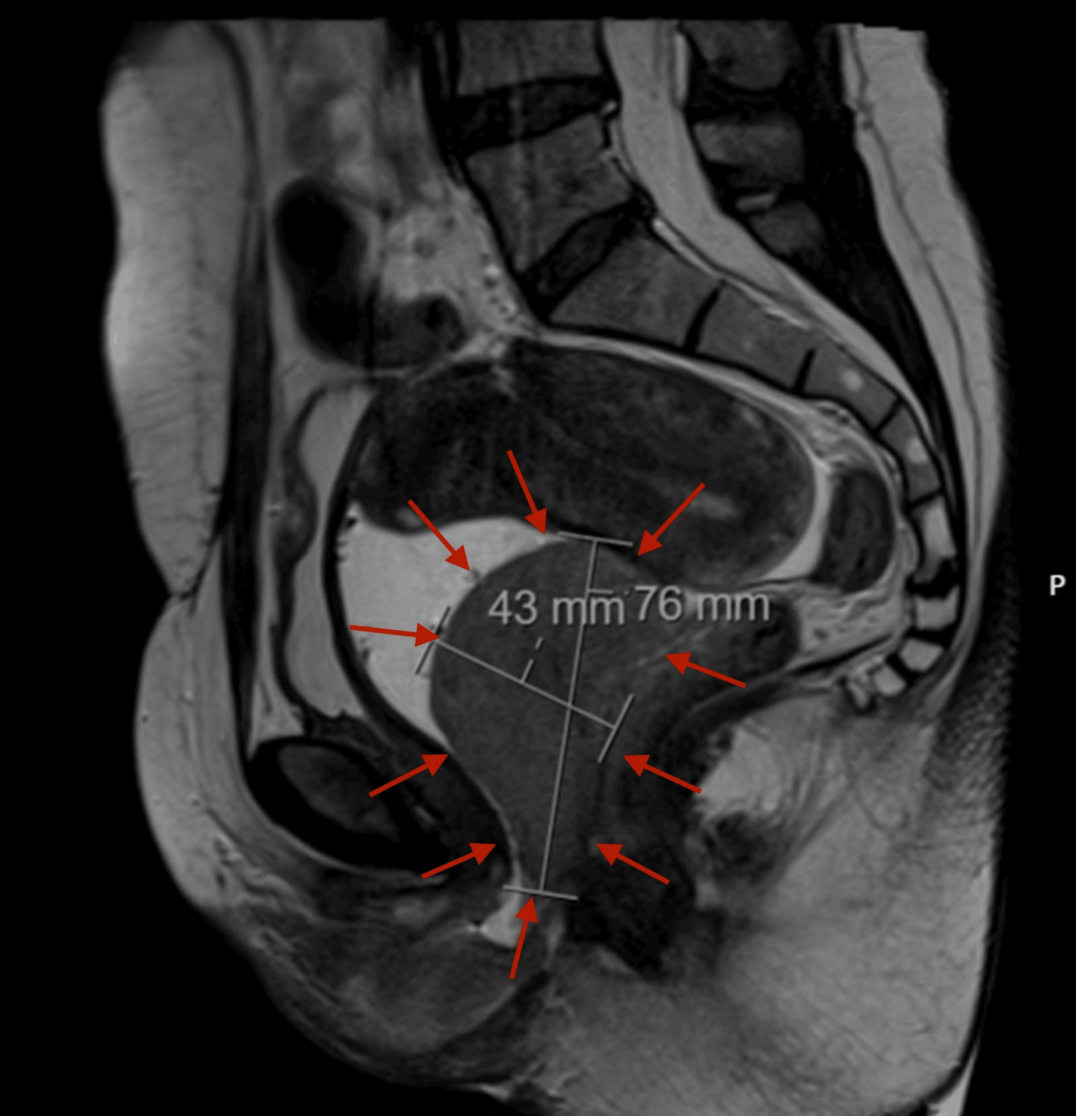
Sagittal pelvic MRI showing a solid mass occupying the rectovaginal space Sagittal T2-weighted pelvic magnetic resonance imaging (MRI) demonstrating a well-defined solid mass measuring 76 × 43 mm (indicated by red arrows) arising from the posterior vaginal wall and extending superiorly toward the cervix. The lesion caused anterior compression of the bladder and posterior displacement of the rectum. During the MRI examination, the vaginal cavity was filled with ultrasound gel to better delineate the lumen, which is clearly visible anterior to the mass. This finding confirms that the lesion originates posterior to the vaginal lumen, consistent with an extramedullary myeloid sarcoma.
